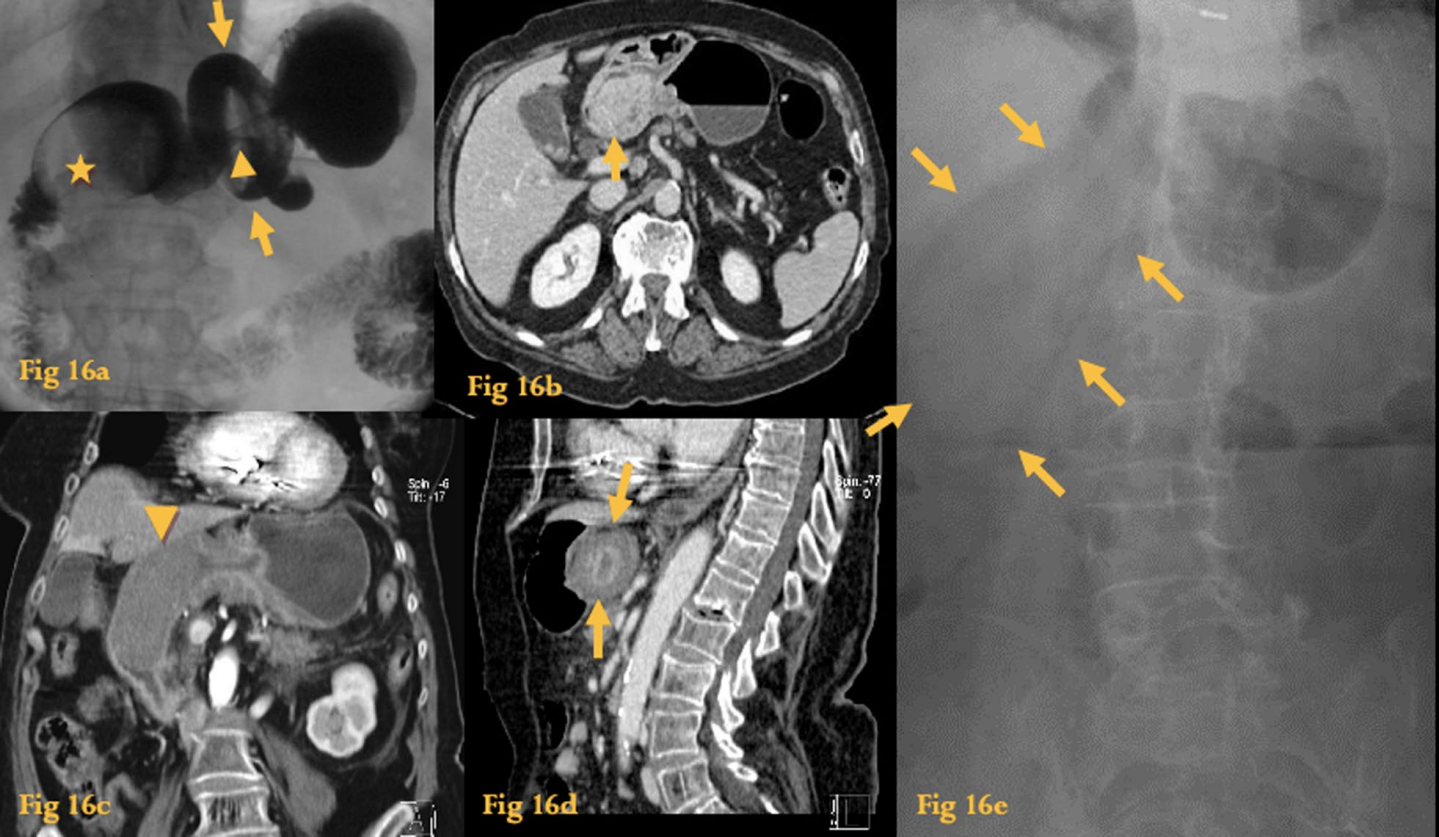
Intussusception. UGIS (a) rounded filling defect in the lumen of the duodenal bulb (asterisk), with regular outlines. The telescoping of mucosal folds (arrowhead) and the prepyloric collar-shaped outpouching (arrows) indicates this is an intussusception of a tumor from the gastric antrum. Abdominal CT (b) of the same patient with a prolapsing polypoid soft-tissue density mass with slightly heterogeneous enhancement (arrow). This was a gastric GIST. Abdominal CT of another gastric GIST (c, d) prolapsing to the duodenum (arrowhead). The coronal reformation shows a typical “target-like” appearance (arrows). Abdominal radiograph (e) of the same patient of 9c, d)—Retrospectively, the intraduodenal mass well-delineated by luminal air was already evident (arrows)
